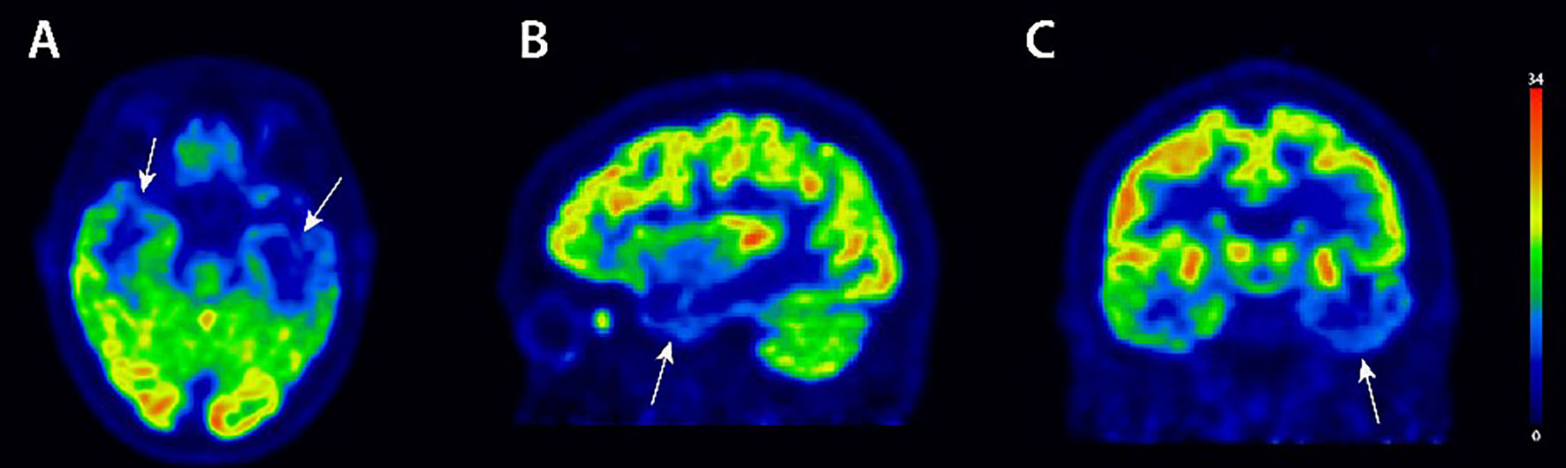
18F-FDG PET/CT imaging. 18F-FDG-PET images in axial (A), sagittal (B) and coronal (C) view showing asymmetric hypometabolism in both temporal lobes (arrows), predominantly in the anterior left temporal lobe. 18F-FDG-PET/CT, 18F-fluorodeoxyglucose (18F-FDG) Positron Emission Tomography (PET)/computed tomography (CT) imaging.
